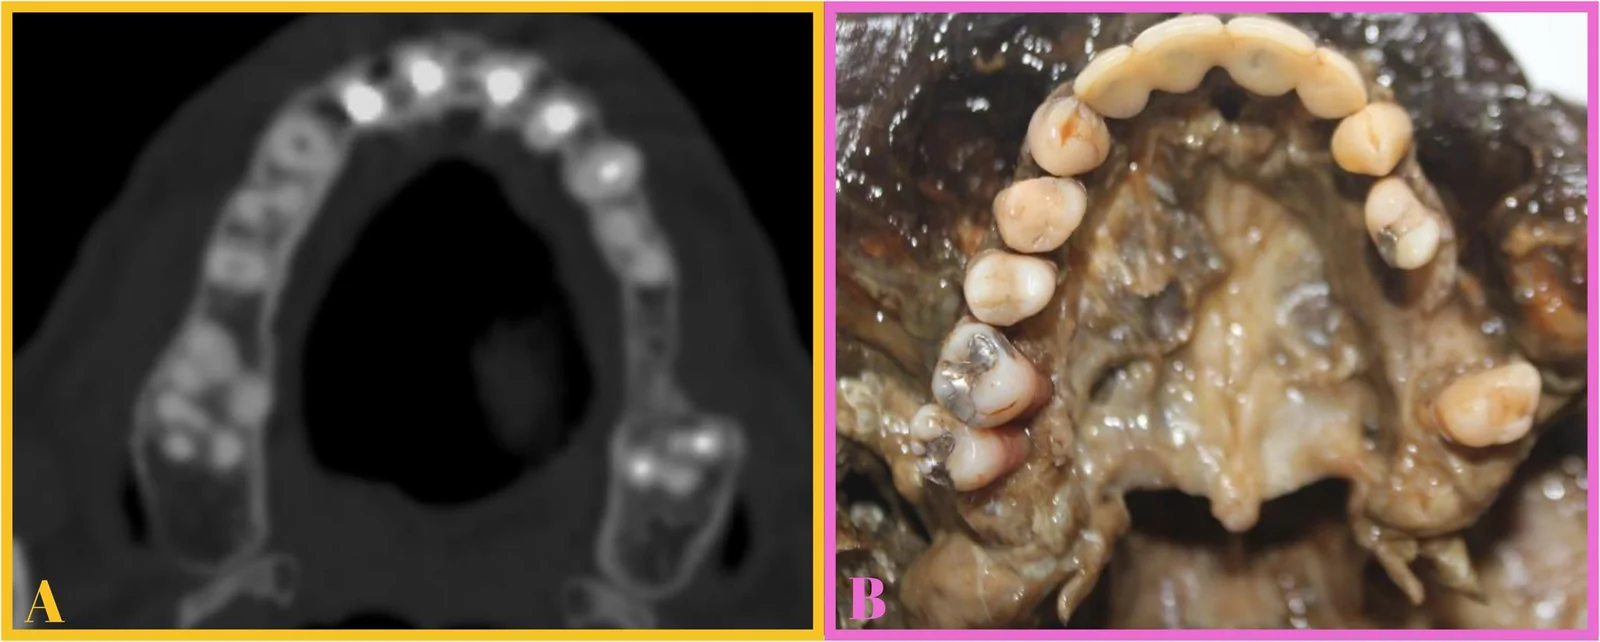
Forensic odontological comparison between an axial CT slice of the maxilla and the occlusal view of the maxillary arch, showing morphological compatibility as well as correspondence between present and absent teeth, their rotational position, and the presence of prostheses on anterior teeth
